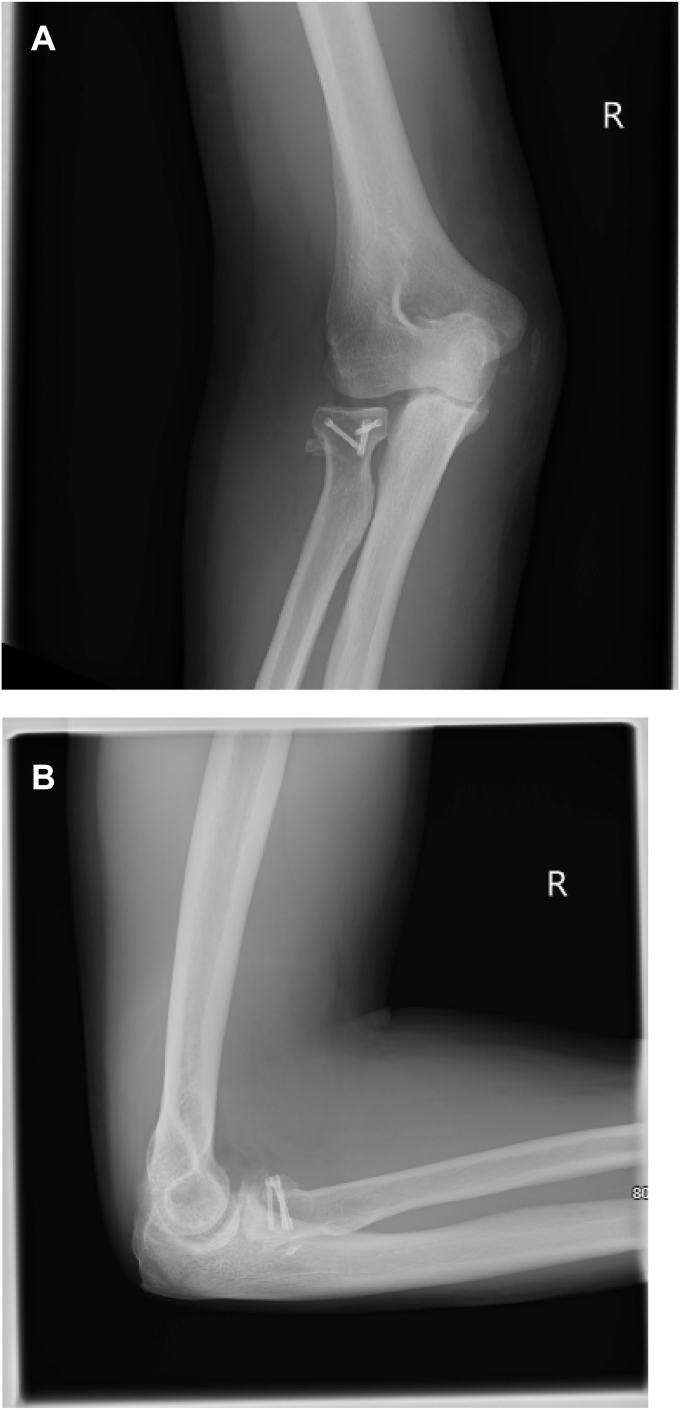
X-rays at one-year postop. (A) Anteroposterior view. (B) Lateral view.
